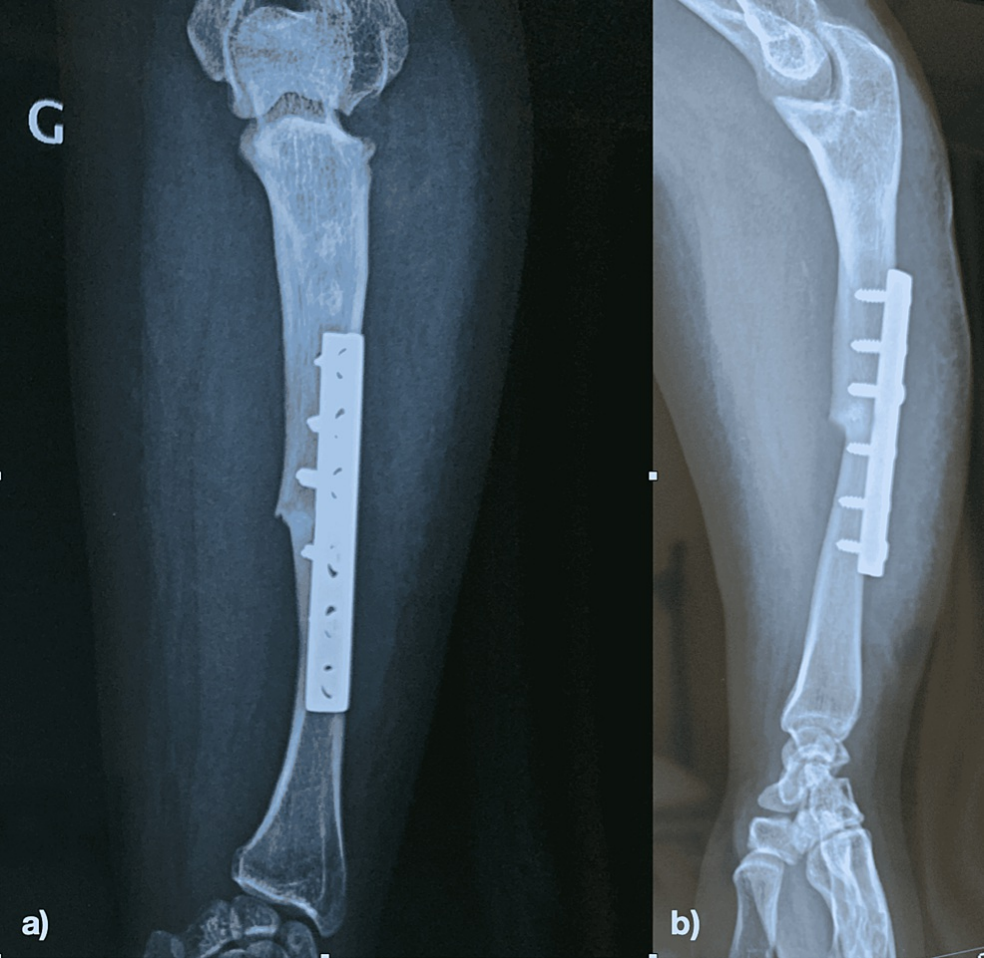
X-ray of the forearm after 4 months: a) Anteroposterior X-ray of the forearm. b) Lateral X-ray of the forearm
